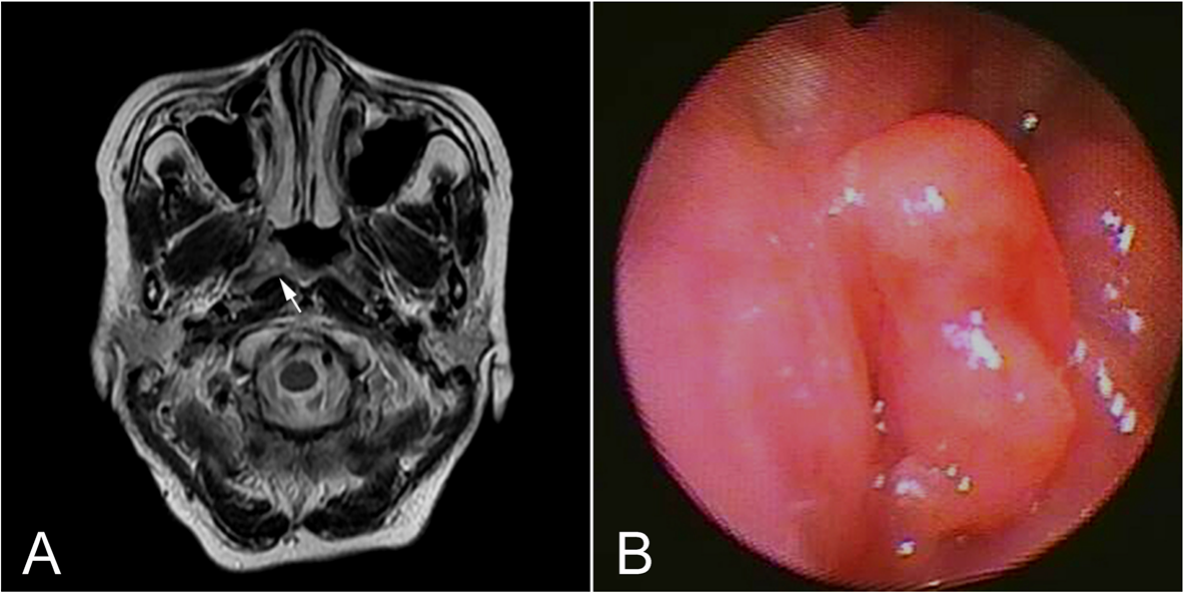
Radiological and nasopharyngeal endoscopic examination. a. MRI revealed a mass protruded from the mucosa of nasopharynx (white arrow). b. Nasopharyngeal endoscopic examination revealed that a smooth well-circumscribed mass extended from the right fossa of Rossenmuller
